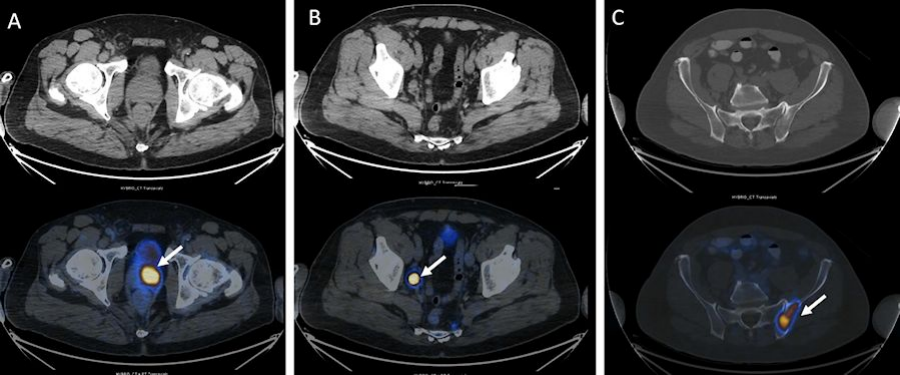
A 59-Year-Old Male with Newly Diagnosed Prostate Cancer (Gleason Score, 7) Staging ^68^Ga PSMA PET/CT scan in a 59-year old male with newly diagnosed prostate cancer (Gleason score, 7) demonstrates pathological PSMA uptake involving most of the left prostate lobe and extending across the midline (A), a right pelvic lymph node metastasis (B), and a left iliac bone metastasis (arrows) (C). PSMA PET is sensitive for detection of micrometastases in nodes less than 1 cm in short axis measurement, as in (C). Additional sites of lymphadenopathy and skeletal metastases were demonstrated on this PSMA PET/CT study (not shown).
